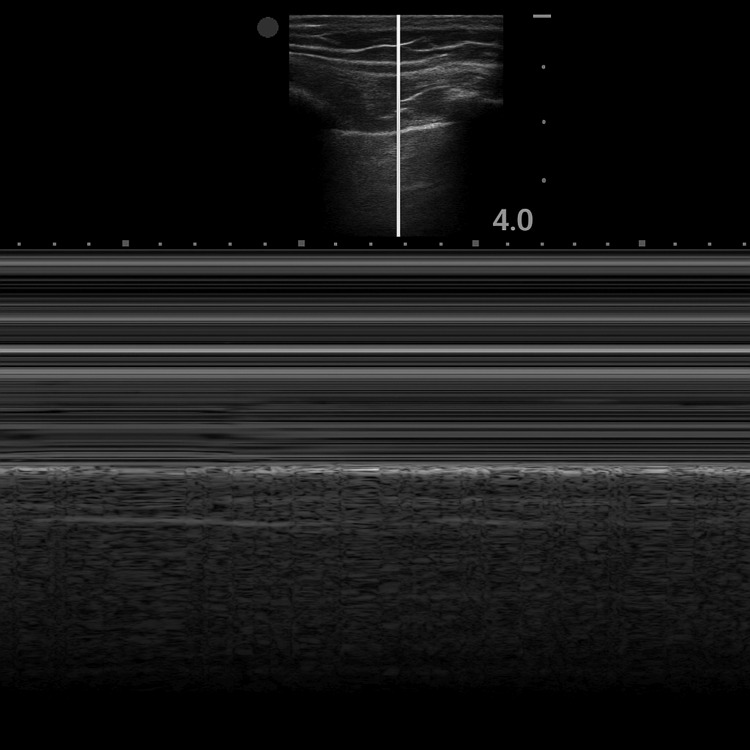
M-mode ultrasound demonstrating the seashore sign in normal lung sliding M-mode lung ultrasound image demonstrating the seashore sign, characterized by parallel horizontal lines in the upper portion of the image (representing the static chest wall) and a granular pattern in the lower portion (representing lung movement). This finding indicates preserved pleural sliding, effectively ruling out pneumothorax at the scanned site.
